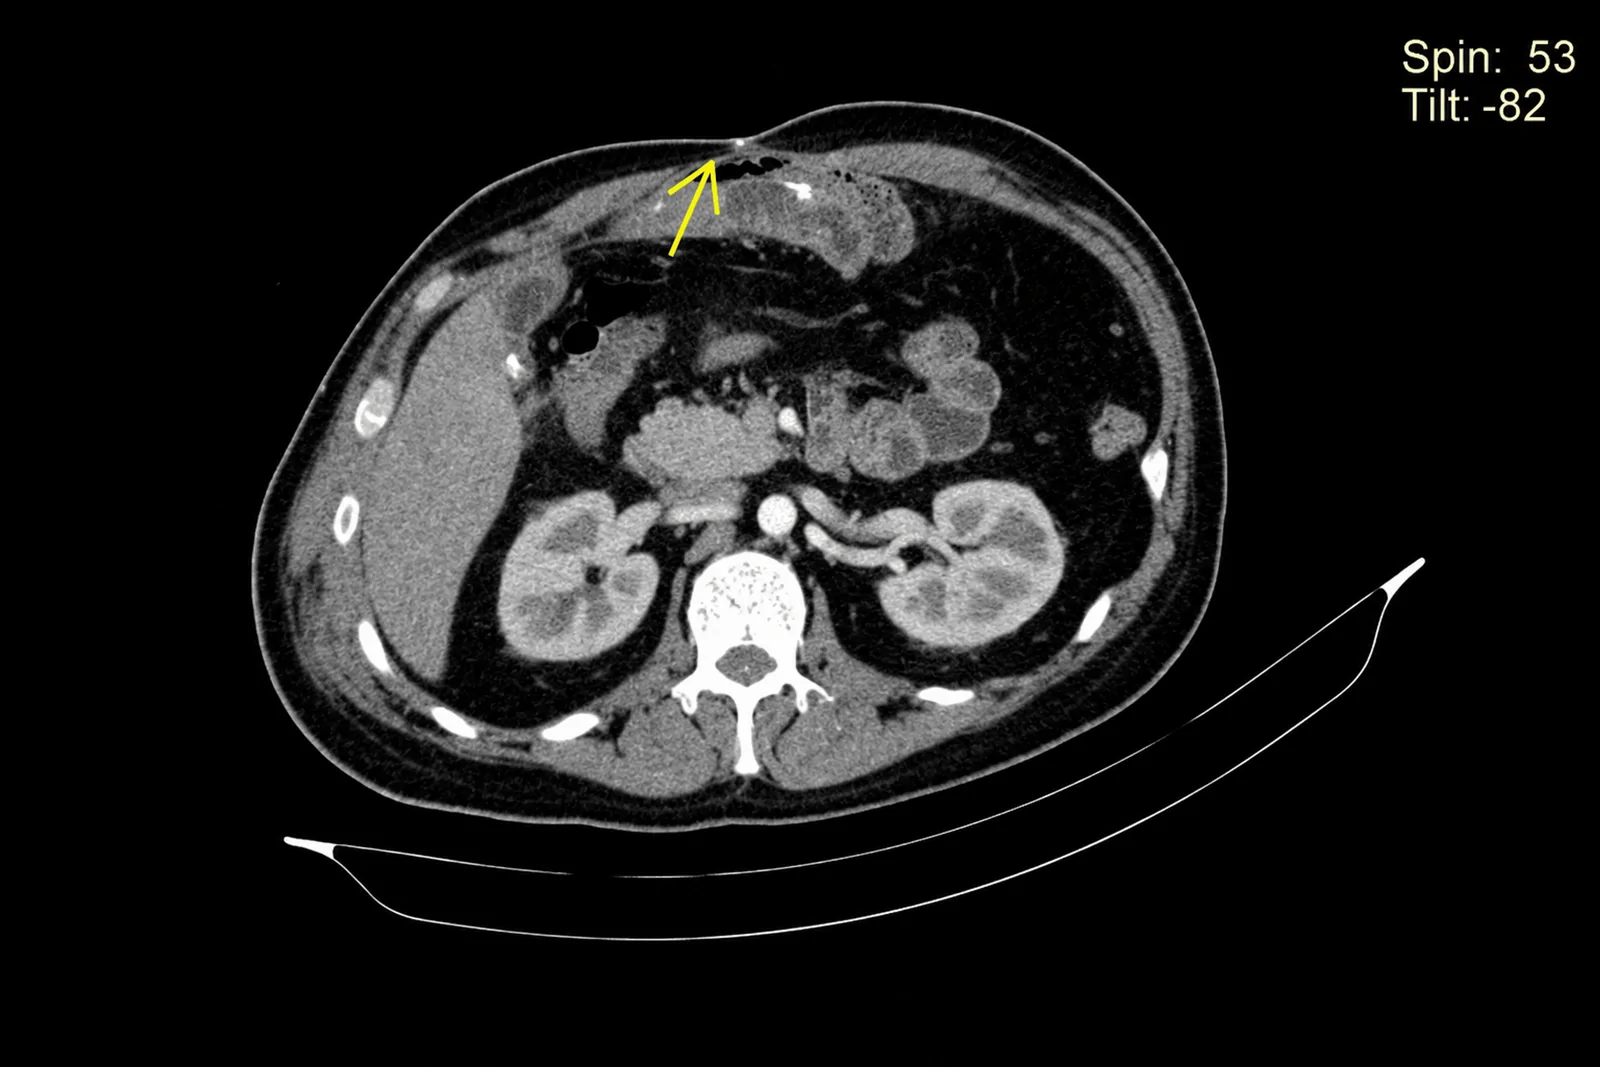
Preoperative CT of the abdominal wall defect. Axial CT image at the level of the left renal hilum shows the upper component of the abdominal wall defect. The arrow shows the precise location of the hernia. CT: computed tomography
